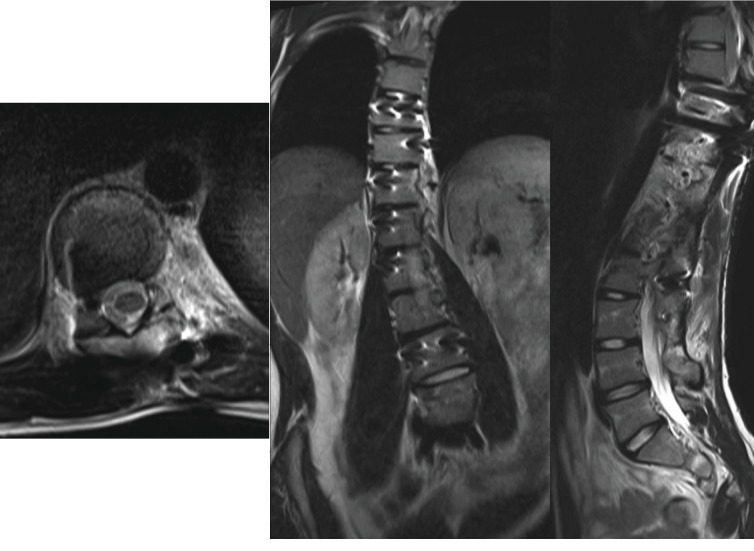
MRI scan at the follow-up visit to outpatient clinic, at July 30^th^, 2018, 2 years and 7 months are passed since the surgery. No tumor growth was detected on the follow-up scan. (a) Axial view at the T11 vertebral body level. The tumor is still confined to pedicle and left paravertebral muscular area. (b) Coronal view. The tumor is still located along the left sides of T10–T12 vertebrae. (c) Sagittal view.
